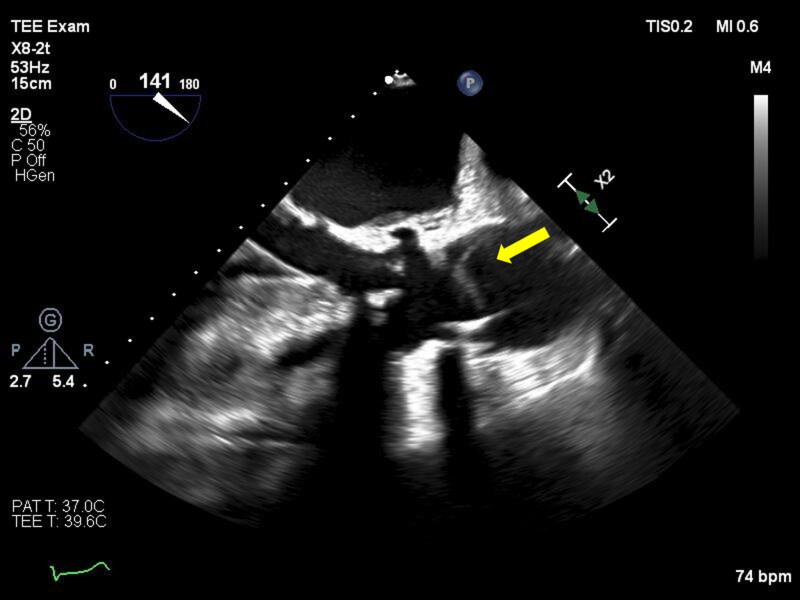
Intraoperative transoesophageal echocardiography in Case 2 (mid-oesophageal aortic valve long-axis view) demonstrates a newly developed intimal flap (arrow) and false lumen in the ascending aorta immediately after deployment of the second valve.
